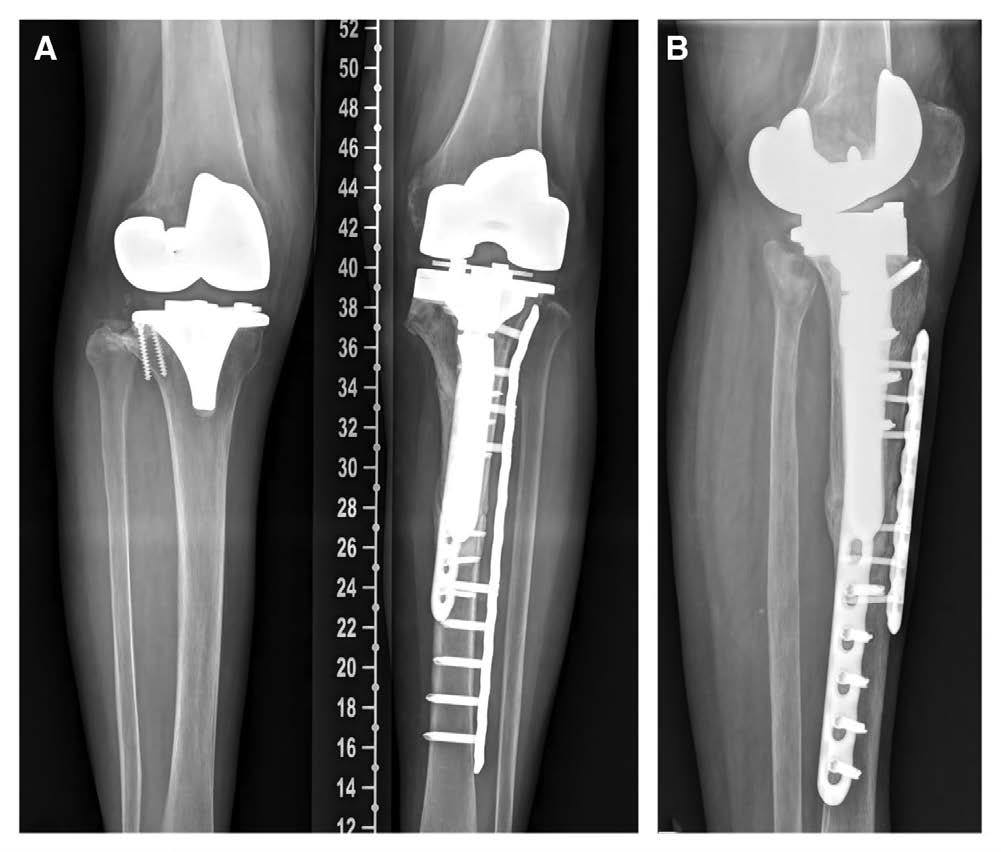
Until now, the patient has had no recurrent PPF, and the fracture is healing from the last X-ray.
